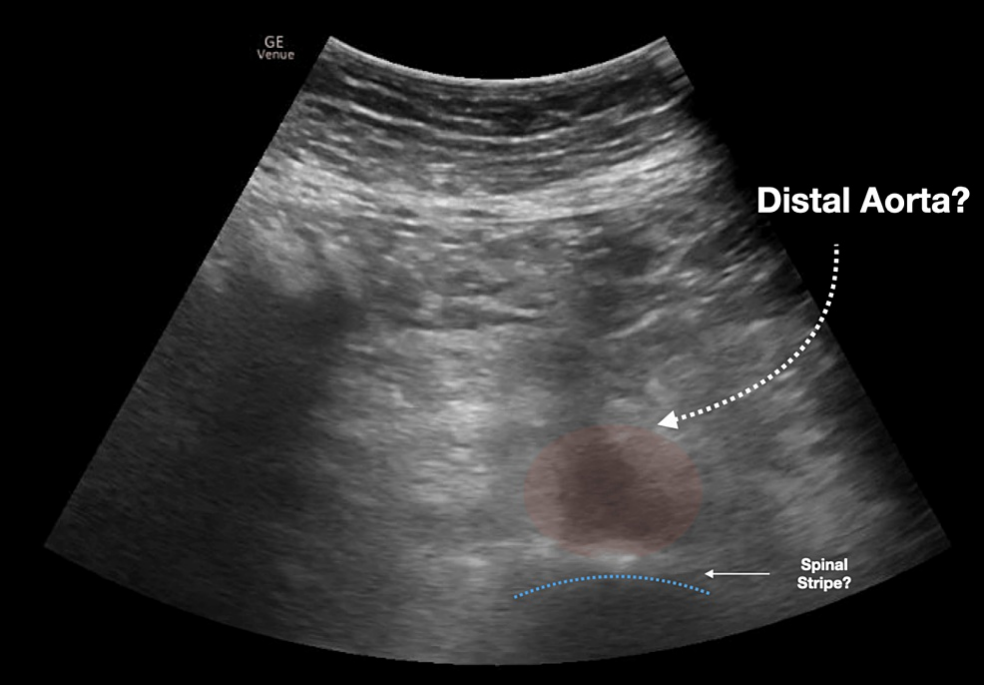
Difficult to visualize the distal aorta In this ultrasound image, shadowing and other artifacts make it difficult to visualize the distal aorta.
